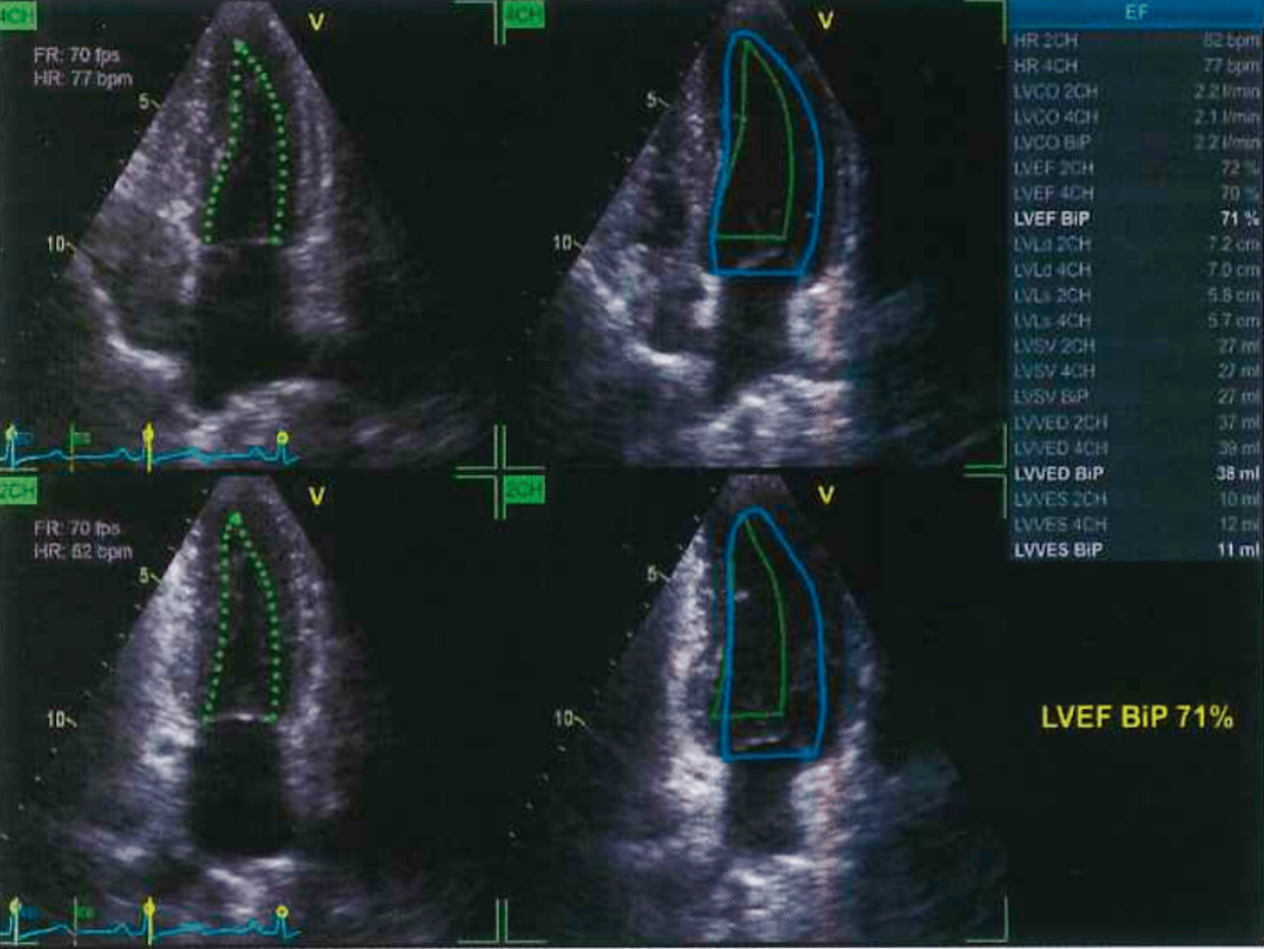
Follow-up TTE six months after initiation of intermediate-dose SAC/VAL and AVF closure, demonstrating recovery of LVEF to 71% and reduction of PASP to 23 mmHg AVF, arteriovenous fistula; LVEF, left ventricular ejection fraction; PASP, pulmonary artery systolic pressure; SAC/VAL, sacubitril/valsartan; TTE, transthoracic echocardiography
